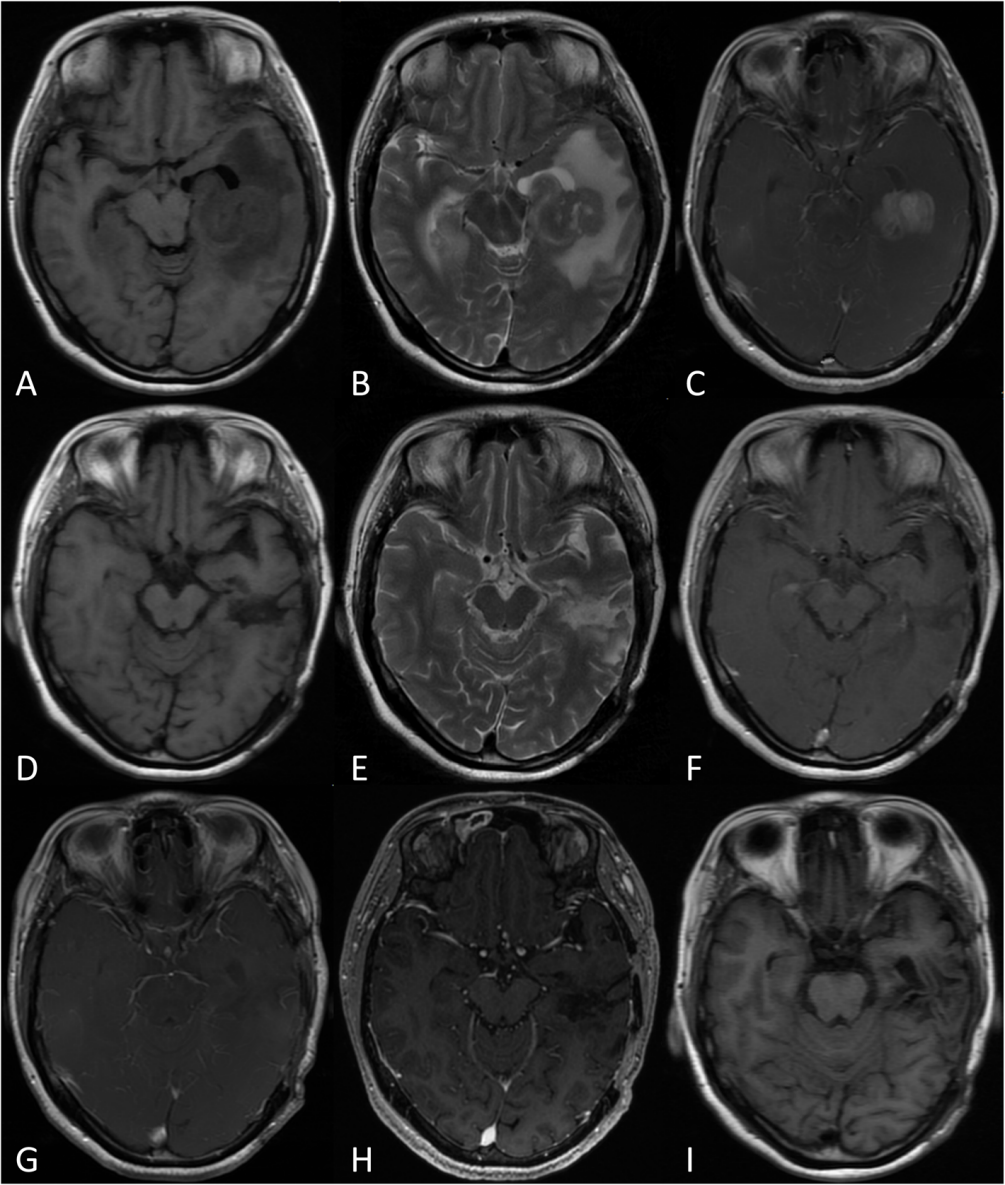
A 42-year-old men who presented with headache for 1 month. MRI (a, b and c) shows a contrast-enhancing solitary lesion with a diameter of 3.5 cm in the left temporal lobe. He underwent surgery with a craniotomy and gross total resection. The symptoms of headache were improved obviously. MRI examination (d, e and f) was taken at 1 week after surgery, which shows the lesion was removed completely. And MRI of 3 months (g), half a year (h) and 1 year (i) after surgery show that there was no recurrence of the tumor
